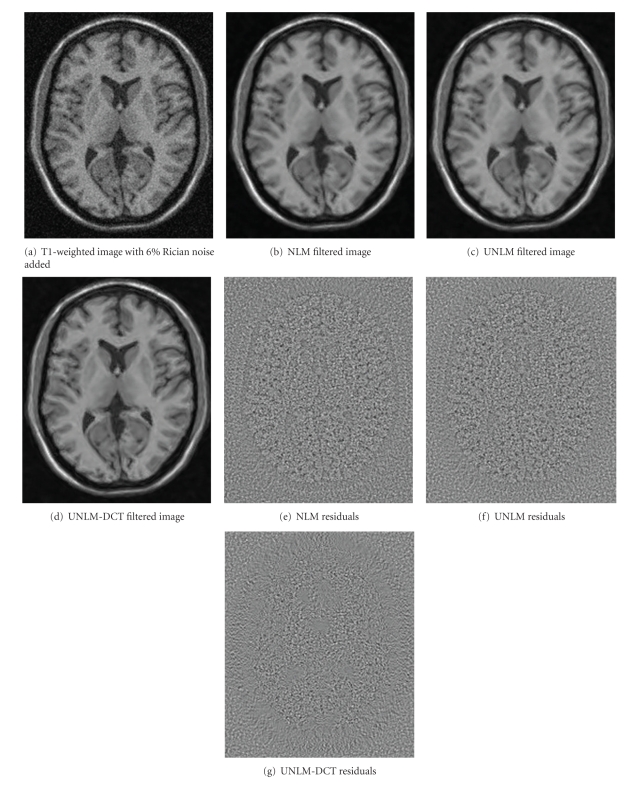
Qualitative comparison of experiment results on 6% Rician noise corrupted T1-weighted image. The quality of the proposed filter can be noticed in both filtered image and the corresponding residuals.
